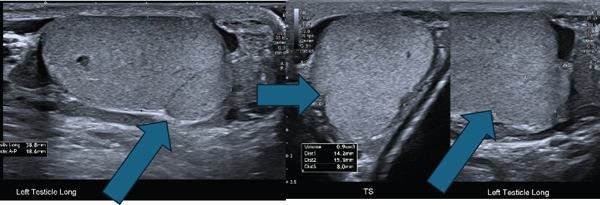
The left testicle measures 35 × 19 × 30 mm, 12 cc. On ultrasonography, it demonstrates a well‐defined, ovoid and solid nodule measuring 14 × 15 × 8 mm with identical echogenicity and internal architecture to the adjacent testicular parenchyma. Arrows demonstrate the ridge between each lobe of the left testicle.
